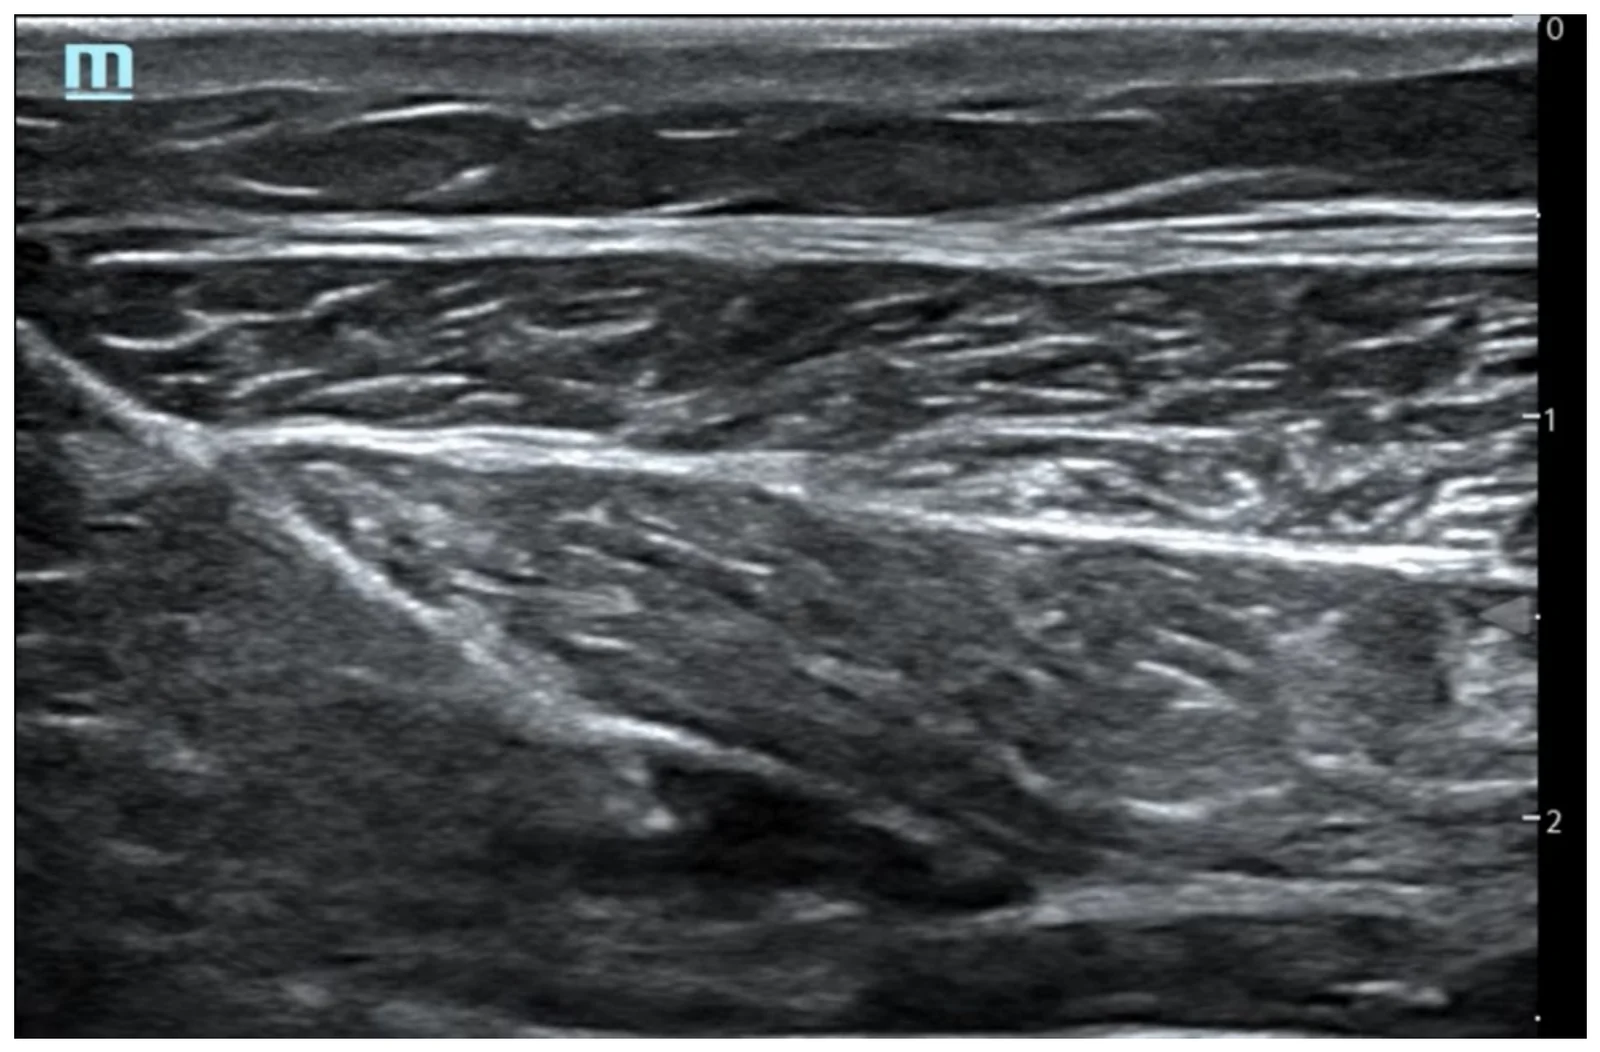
Ultrasound view of hydrodissection during TAPB.
